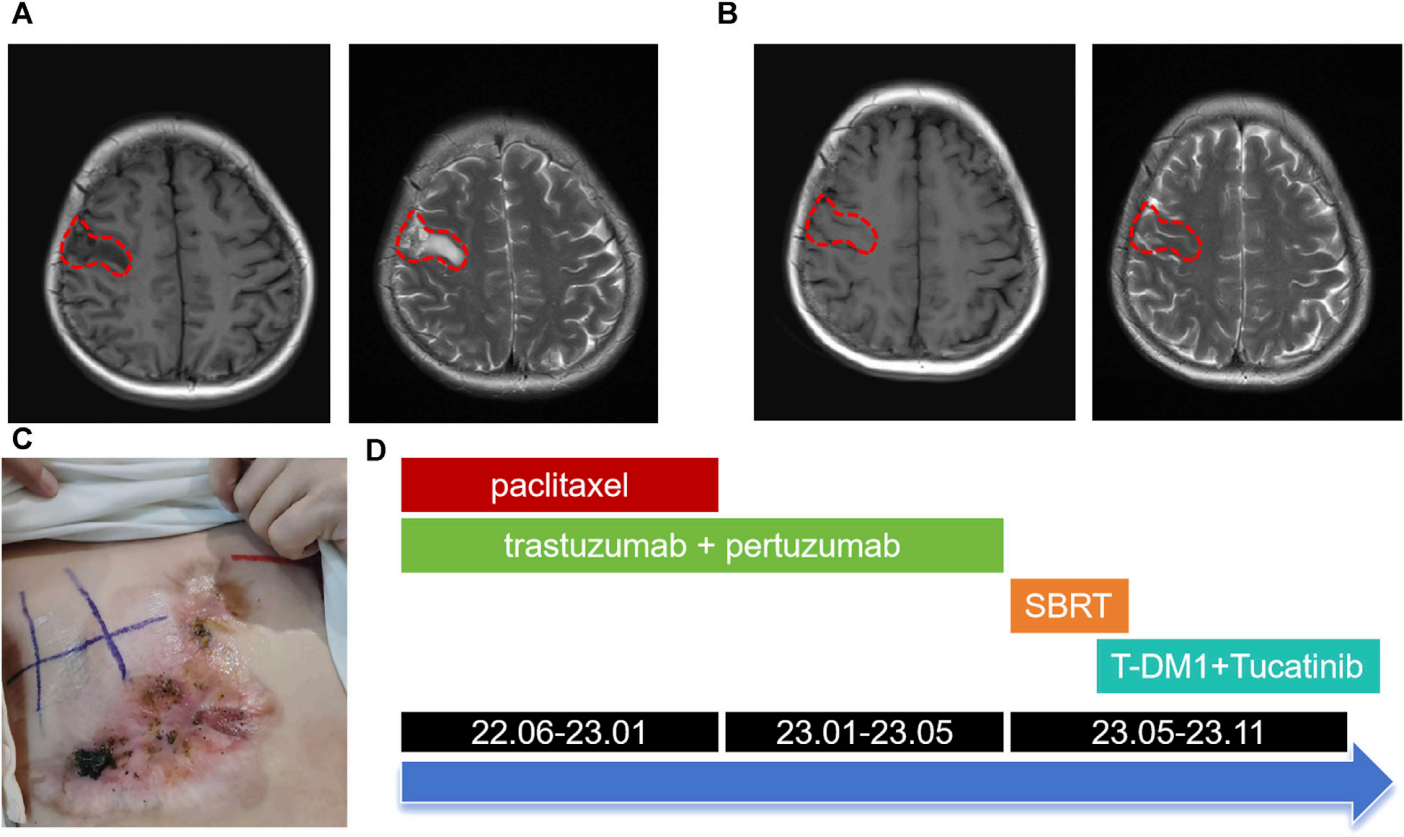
Brain MRI images and the overall treatment timeline. (A) Brain MRI images at May 2023 showed right frontal lobe nodule accompanied by edema in the adjacent brain tissue (lesions outlined with red dashed lines). (B) Brain MRI images after SBRT and combined therapy including T-DM1 combined with Tucatinib. (C) Changes in the right chest mass appearance at October 2023. (D) Treatment timeline from June 2022 to November 2023.
